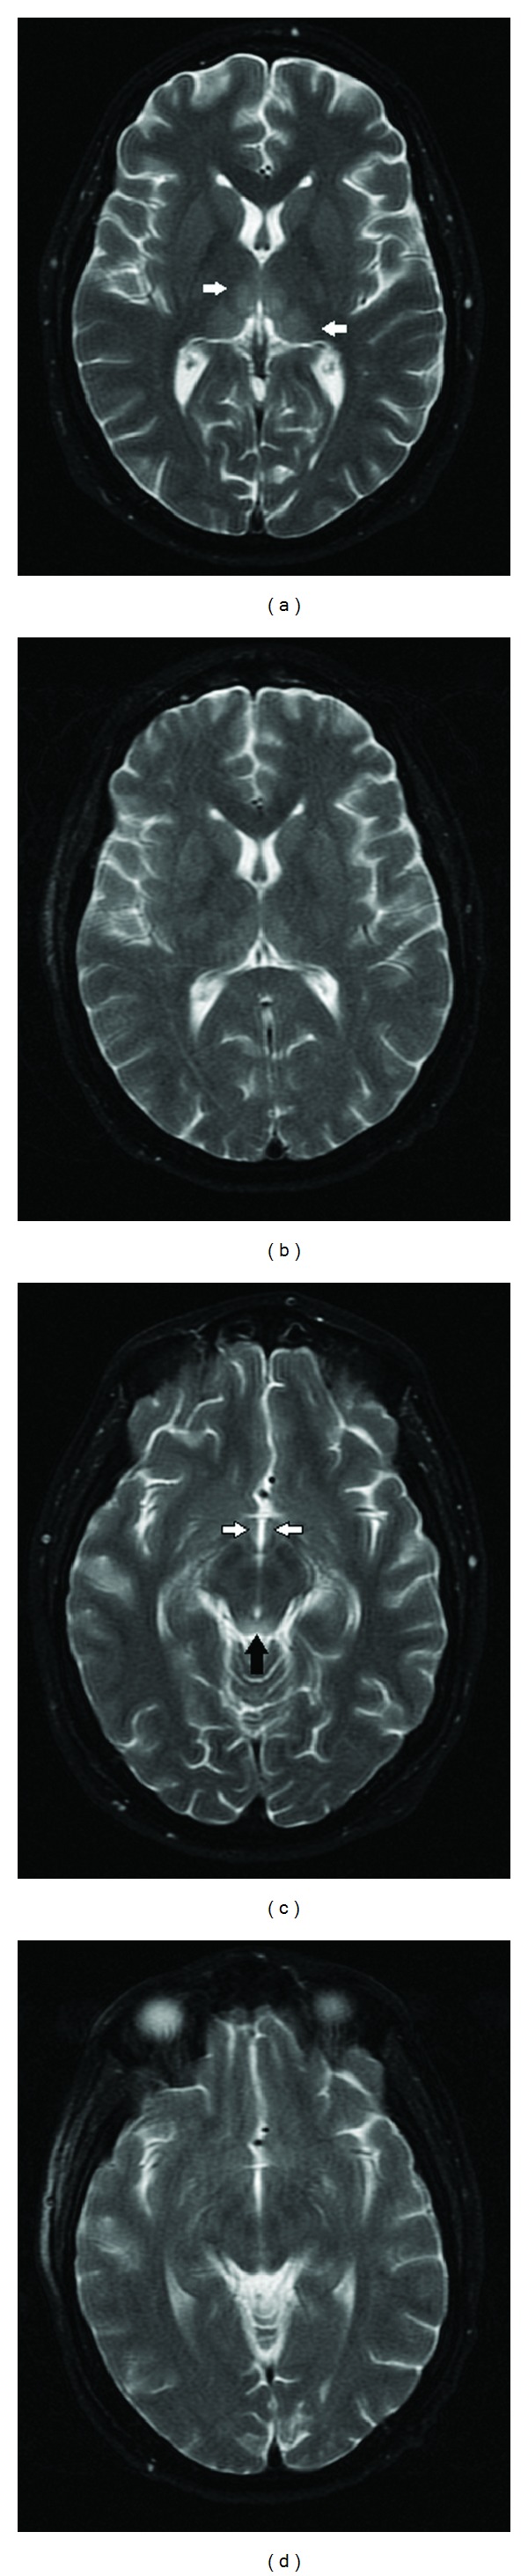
T2 brain MRI one day ((a), (c)) and seven days ((b), (d)) after admission. Signal abnormalities in bilateral thalami ((a), arrows) and interval improvement (b). Signal abnormalities involving the hypothalamus ((c), white arrows), periaqueductal gray matter ((c), black arrows), and interval improvement (d).
